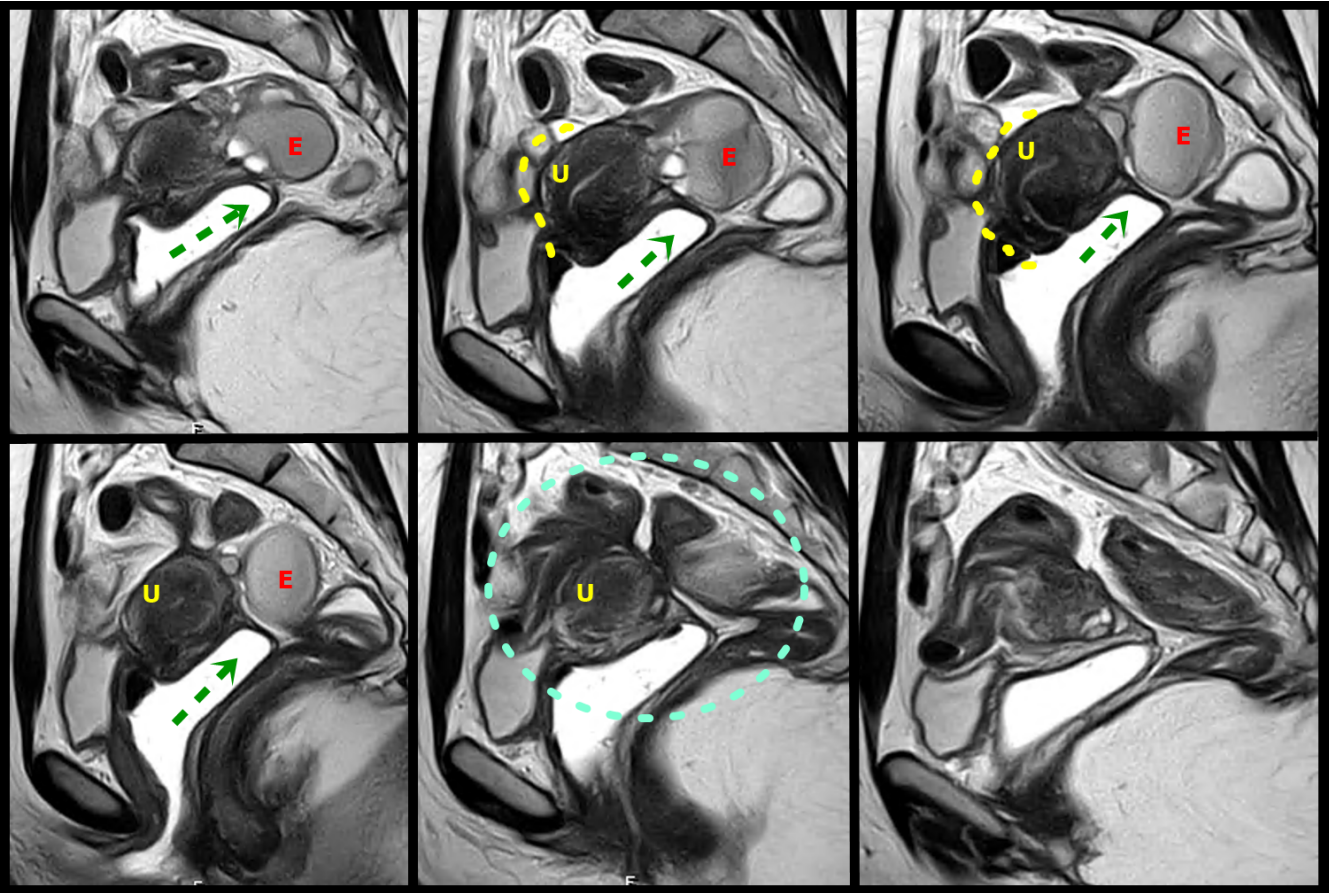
Sagital T2W MR images with distension of the vagina by aqueous gel show a plaque in the rectouterine and retrocervical space, invading and elevating of the posterior vaginal fornix (green arrow), causing obliteration of the posterior cul-de-sac (green circle). The lesion infiltrated the posterior uterine serosa and myometrium and is causing uterine retractile retroflexion (yellow interrupted line)A left adnexal endometrioma (E) demonstrates “T2 shading” and associated fibrotic change. U uterus.
